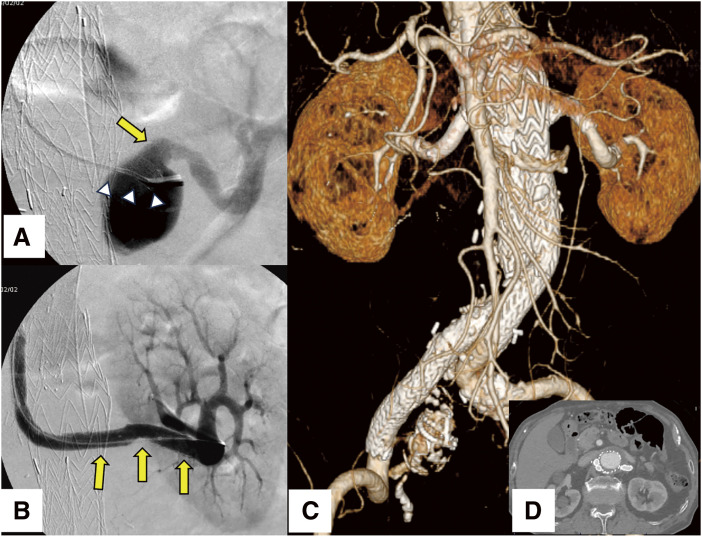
Fig. 3 (A) Angiography reveals a pronounced gap between the renal chimney stent graft (arrowheads) and the renal artery ostium (arrow), attributable to the enlarged aneurysmal sac. (B) Angiography confirms complete resolution of the endoleak following the deployment of a stent graft to bridge the gap between the chimney stent graft and the left renal artery (arrows). (C) CTA demonstrates no evidence of further stent graft migration or endoleak during a 3-year follow-up period. (D) Axial imaging demonstrates regression of the aneurysm. CTA: computed tomography angiography
